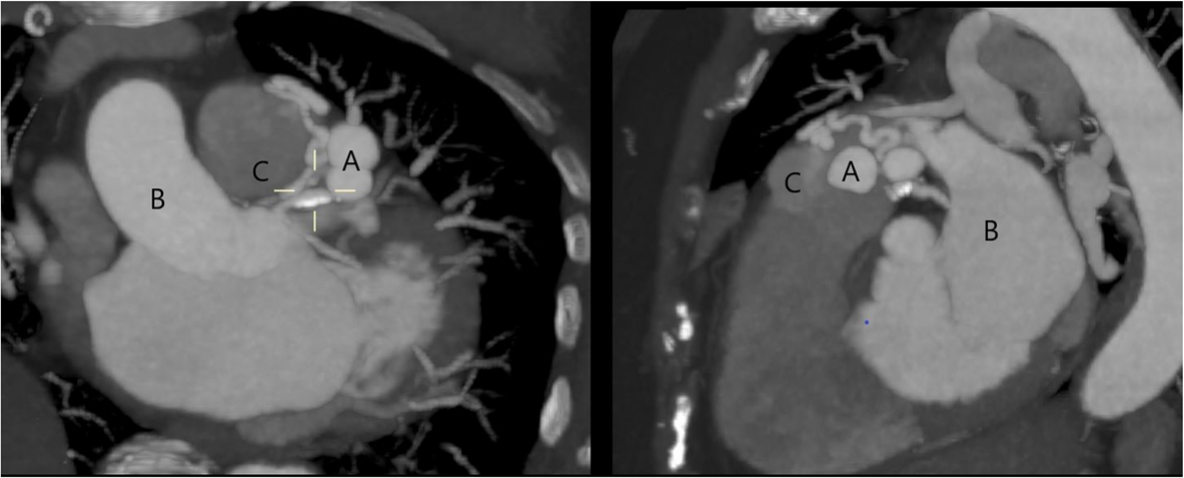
Multiplanar reformat (MPR) images of the coronary CTA demonstrating: a inflow aneurysms from LADD and artery fistula, b aorta, c pulmonary artery with a subtle contrast blush as a result of abnormal communication from LADD to PA
